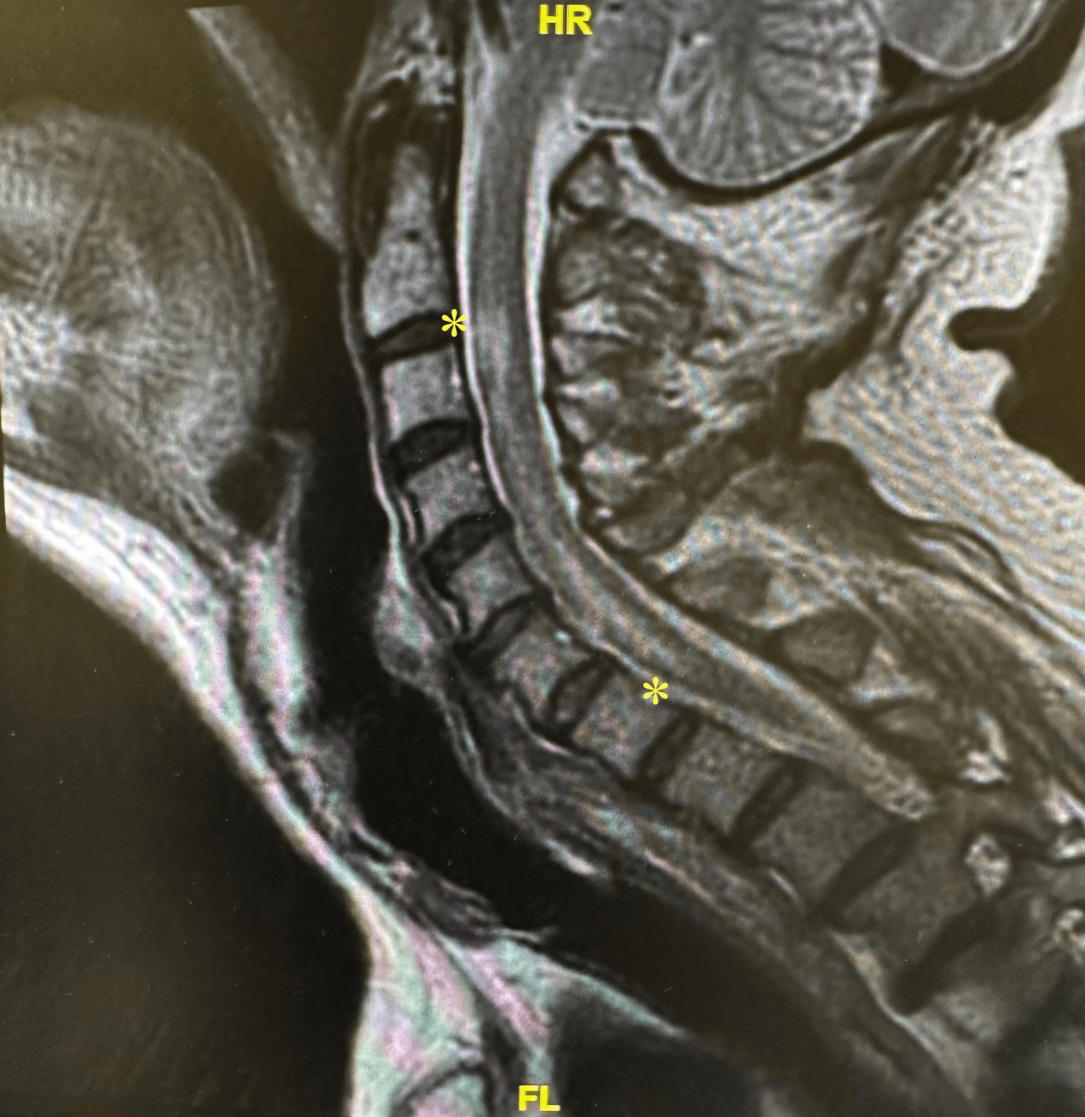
MRI with asterisk showing a diffuse T2 from cervical spine two through seven. MRI: magnetic resonance imaging.
